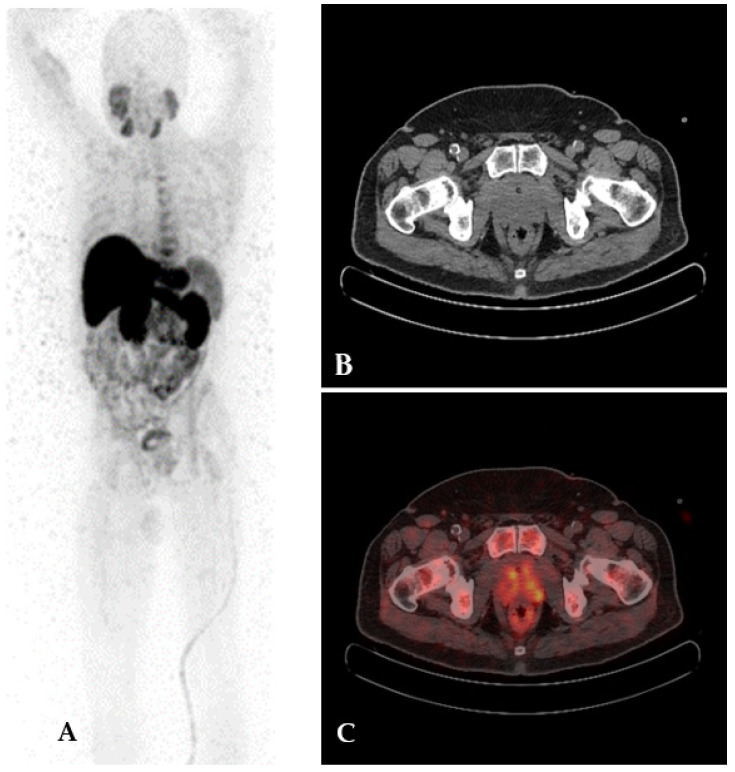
^18^F-choline PET/CT imaging in a patient with prostate cancer. Maximum intensity projection (A), CT (B), and fused (C) axial slices show increased tracer uptake in the upper lobes of the prostate.
